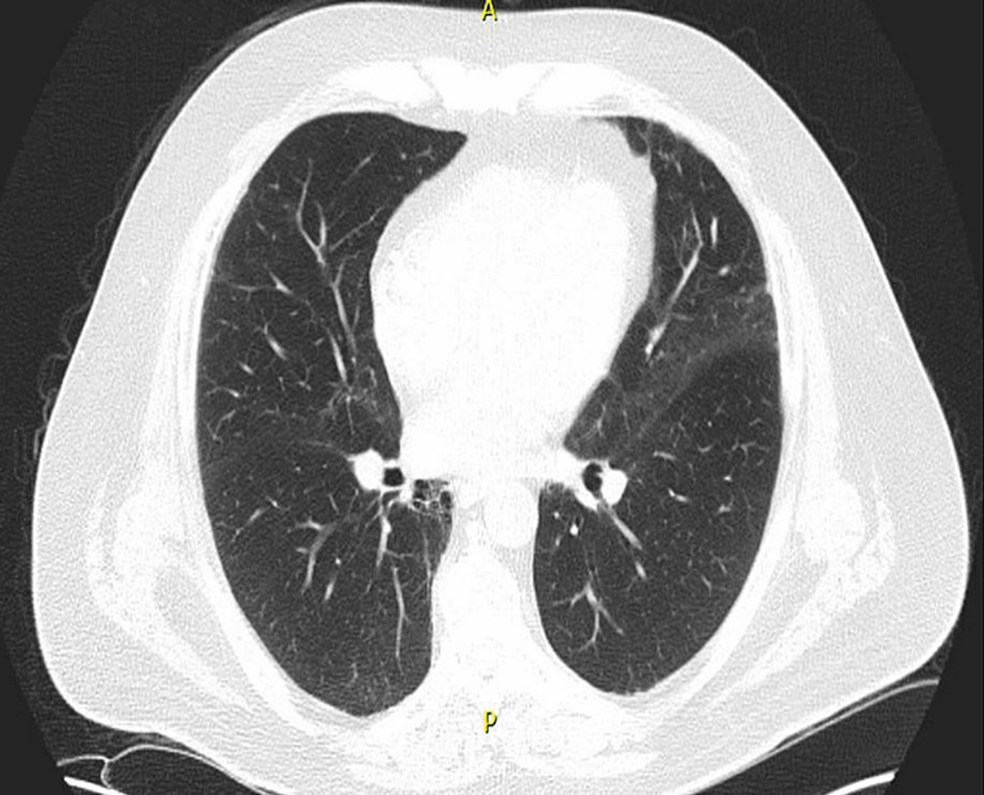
High-resolution CT findings at the time of GPA diagnosis The image shows no signs of interstitial lung disease, bronchiectasis, or consolidation CT: computed tomography; GPA: granulomatosis with polyangiitis
